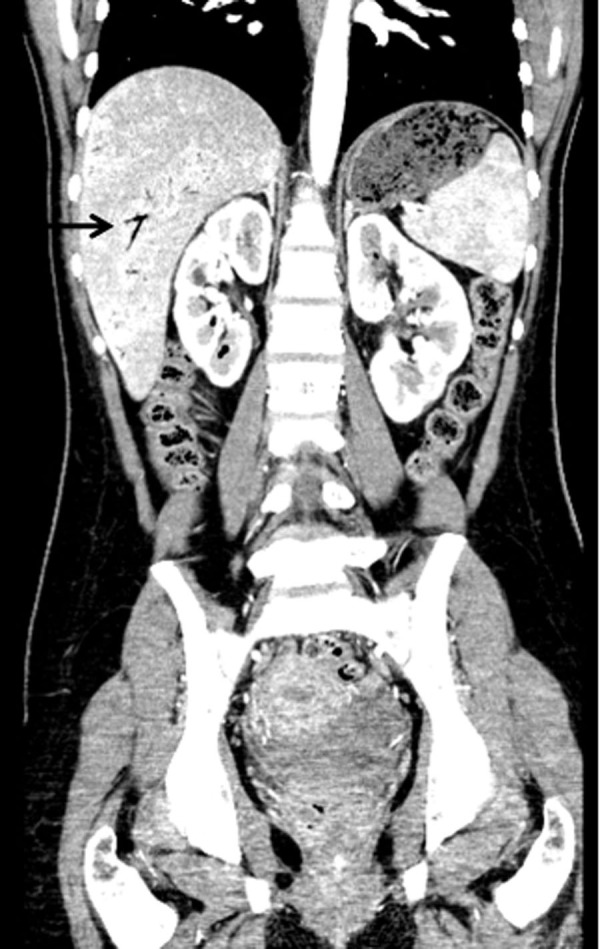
Coronal view of abdominal CT with contrast showing air in the collecting system of both kidneys and air in the portal tract (arrow).
